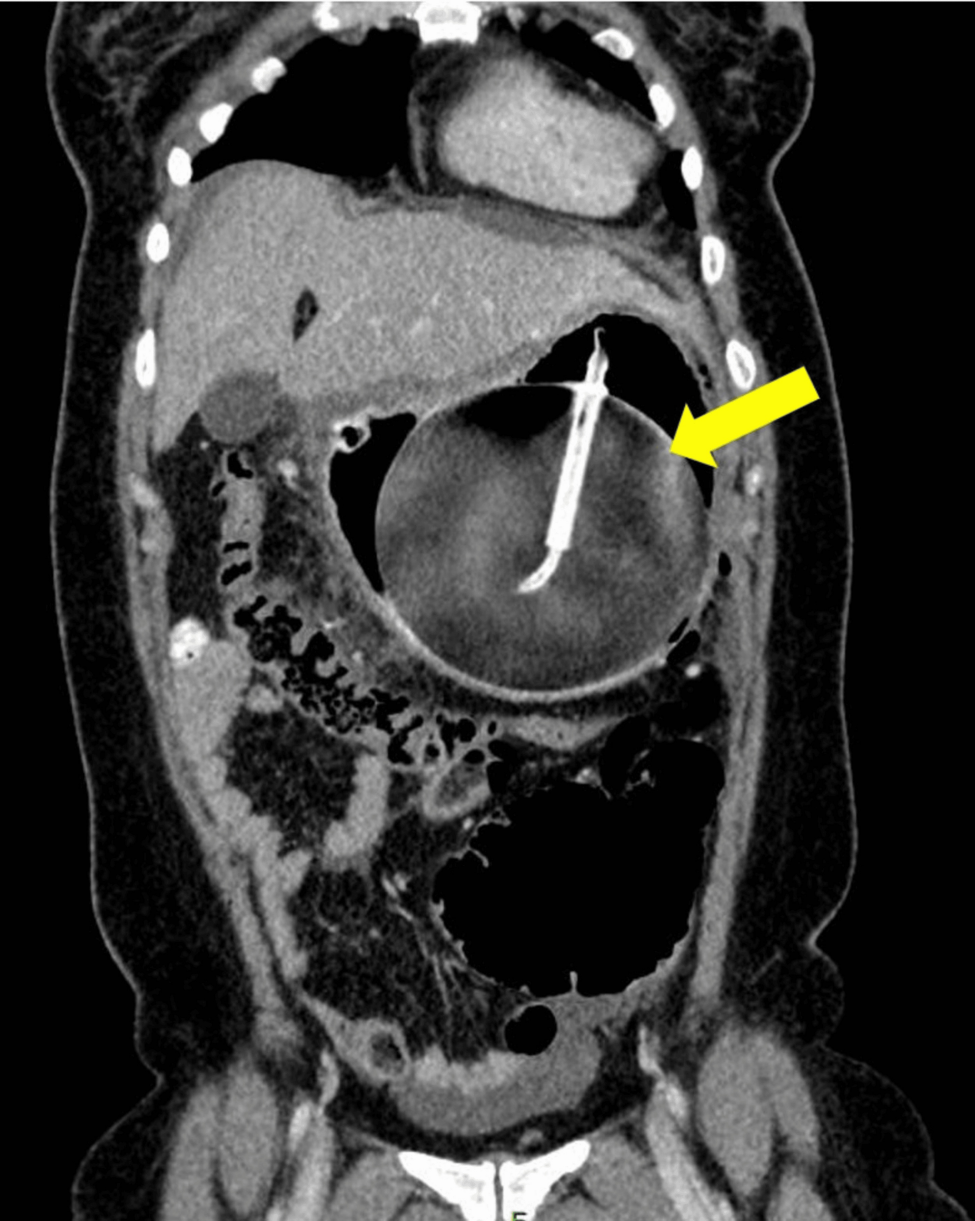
Contrast-enhanced CT of the abdomen (coronal view) showing the hyperinflated intragastric balloon (yellow arrow) within the stomach occupying the entire upper quadrant of the abdomen.
